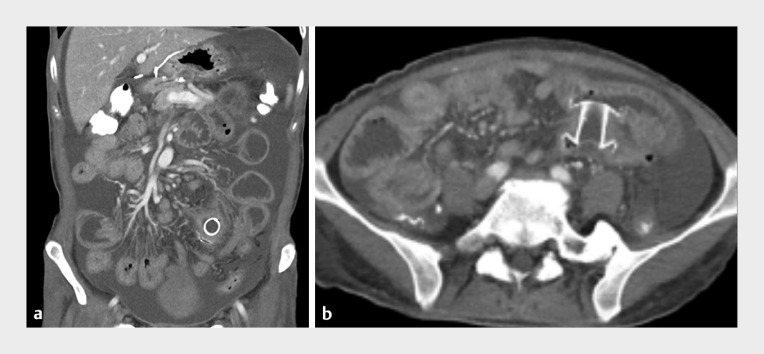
Images from the subsequent computed tomography scan 2 weeks after the endoscopic ultrasound-guided ileocolostomy showing the correctly positioned lumen-apposing metal stent, which has decompressed the entire small intestine.
